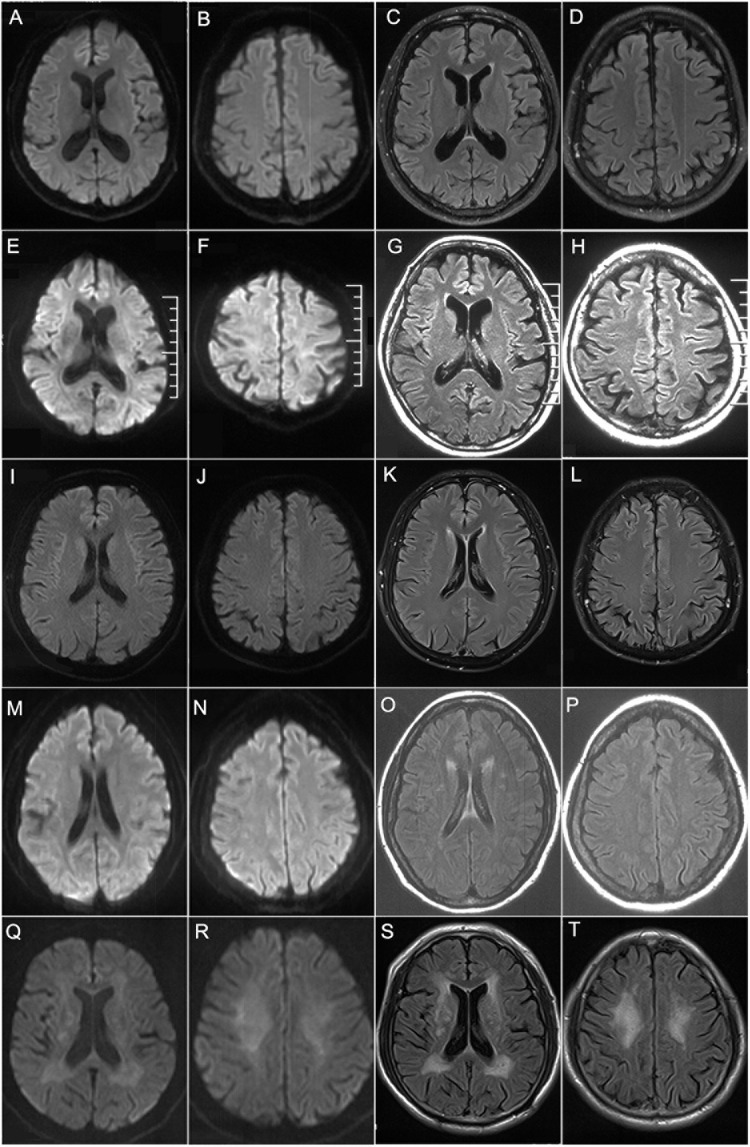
Brain MRI findings. Brain MRIs of patient 1 (A–D), patient 2 (E–H), patient 3 (I–L), patient 4 (M–P), and patient 5 (Q–T). (A–L) showed no obvious abnormalities on DWI (A,B,E,F,I,J) or T2-FLAIR (C,D,G,H,K,L). (O,P,S,T) showed high signals along the lateral ventricle on T2-FLAIR while no obvious abnormalities on DWI (M,N,Q,R).
